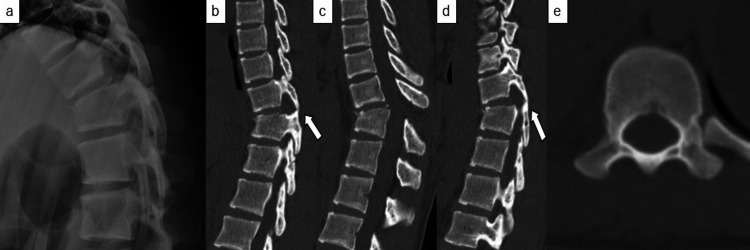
Preoperative imaging examination (a) Preoperative plain thoracic X-ray. There was a compression wedge fracture of T11. (b) Preoperative plain thoracic computed tomography (CT) of the left facet joint. (c) Preoperative plain thoracic CT in the sagittal plane. There was a compression wedge fracture of T11. (d) Preoperative plain thoracic CT of the right facet joint. (e) Preoperative plain thoracic CT in the axial plane
